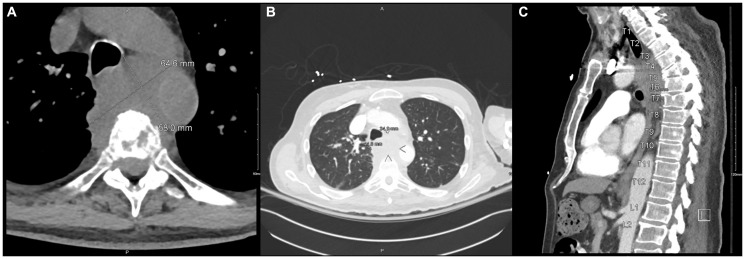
Cross-sectional and sagittal CT images demonstrating the posteriorly invasive esophageal adenocarcinoma. (A) Axial CT showing a 64.6 × 58.0 mm posterior mediastinal mass abutting the esophagus and invading the T5 vertebral body. (B) Axial lung window showing mediastinal lymphadenopathy and right hilar involvement (34.9 mm and 44.8 mm). (C) Sagittal reconstruction revealing extensive T5 vertebral destruction and epidural extension causing spinal canal compromise.
